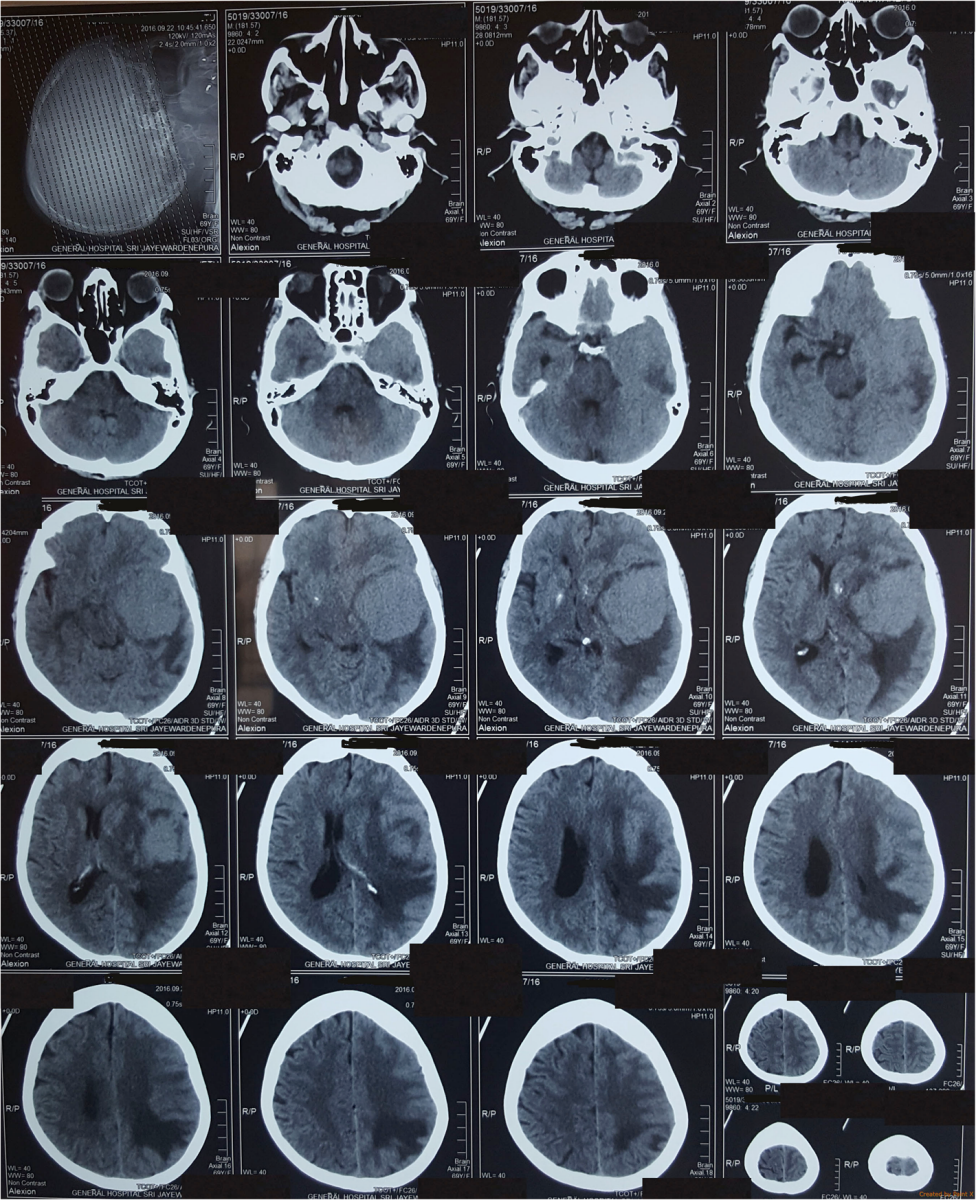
Noncontrast CT scan of the brain revealing two small areas of haemorrhage in the tumour
